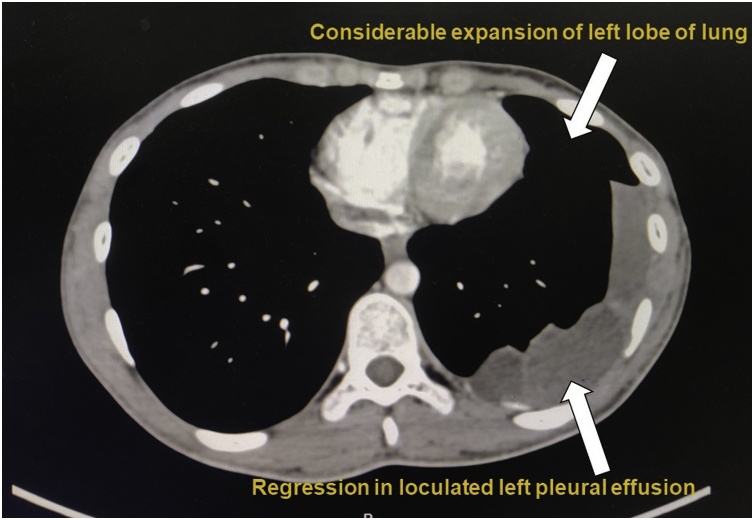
CT chest done 5 months post decortication of lung suggestive of “regression of left pleural effusion with considerable expansion of left lobe of lung”.
